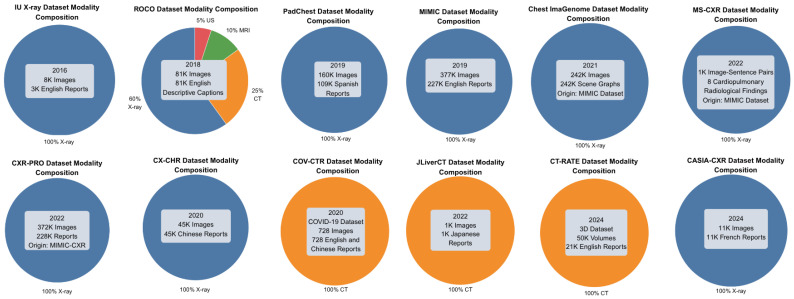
The dataset modality composition. Blue indicates X-ray, orange indicates CT scans, and green indicates MRI.
